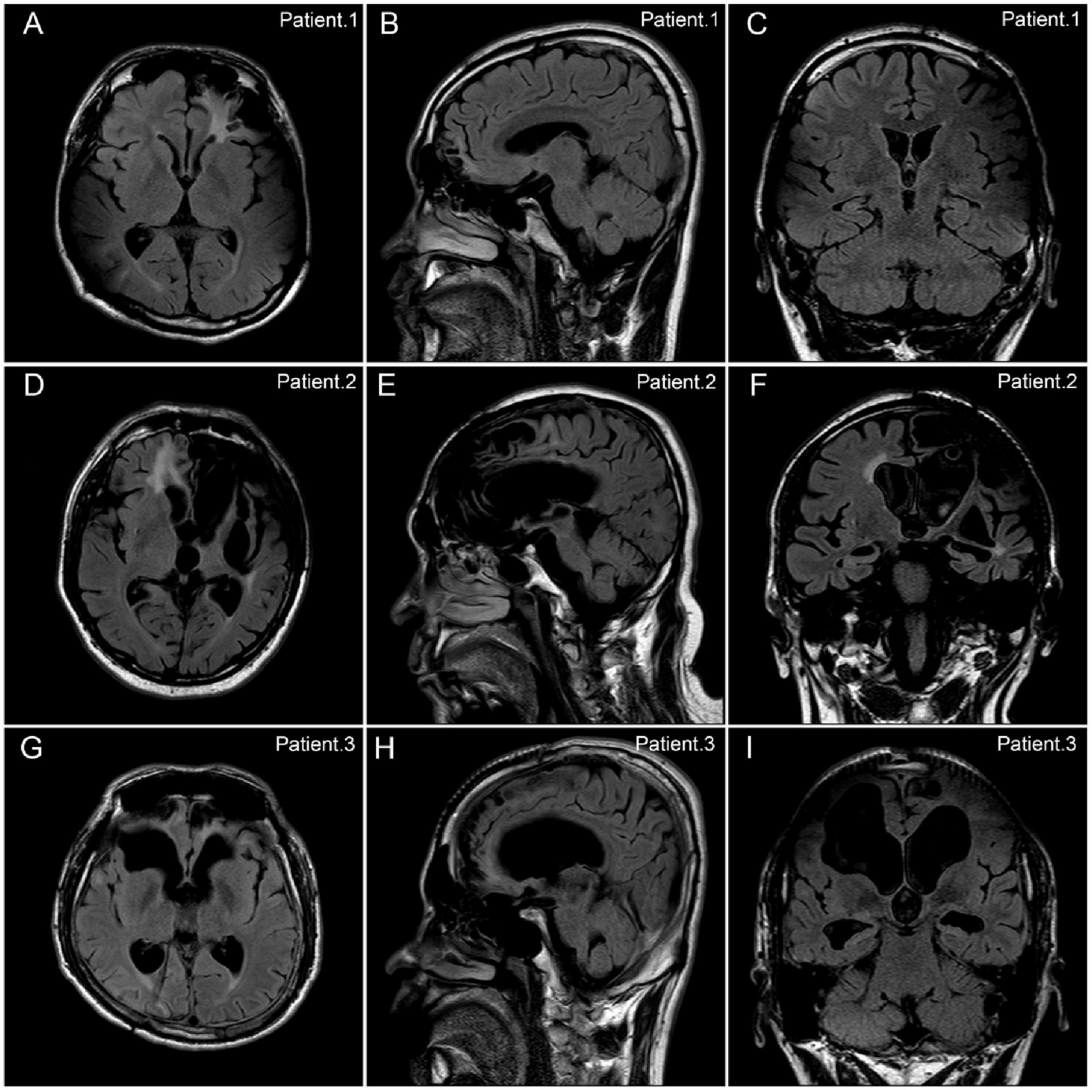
Representative FLAIR MR images of patients with refractory posttraumatic epilepsy. There were representative FLAIR MR images of three patients with refractory posttraumatic epilepsy (PTE) in the axial (A,D,G), sagittal (B,E,H), and coronal (C,F,I) planes. (A–C) Patient No.1. A 22-year-old boy with refractory PTE due to motor vehicle accident. The encephalomalacia was observed in bilateral frontal, parietal, and temporal lobes. The patient got seizure freedom after 2 years following the VNS therapy. (D–F) Patient No.2. A 51-year-old man with refractory PTE due to blunt trauma. The encephalomalacia was observed in the left frontal, temporal, and insula lobes, as well as in the right frontal lobe. The patient got a 75% reduction in seizure frequency after 7.5 years following the VNS therapy. (G–I) Patient No.3. A 26-year-old woman with refractory PTE due to motor vehicle accident. The encephalomalacia was observed in the bilateral frontal lobes. The patient got a 75% reduction in seizure frequency after 8 years following the VNS therapy.
